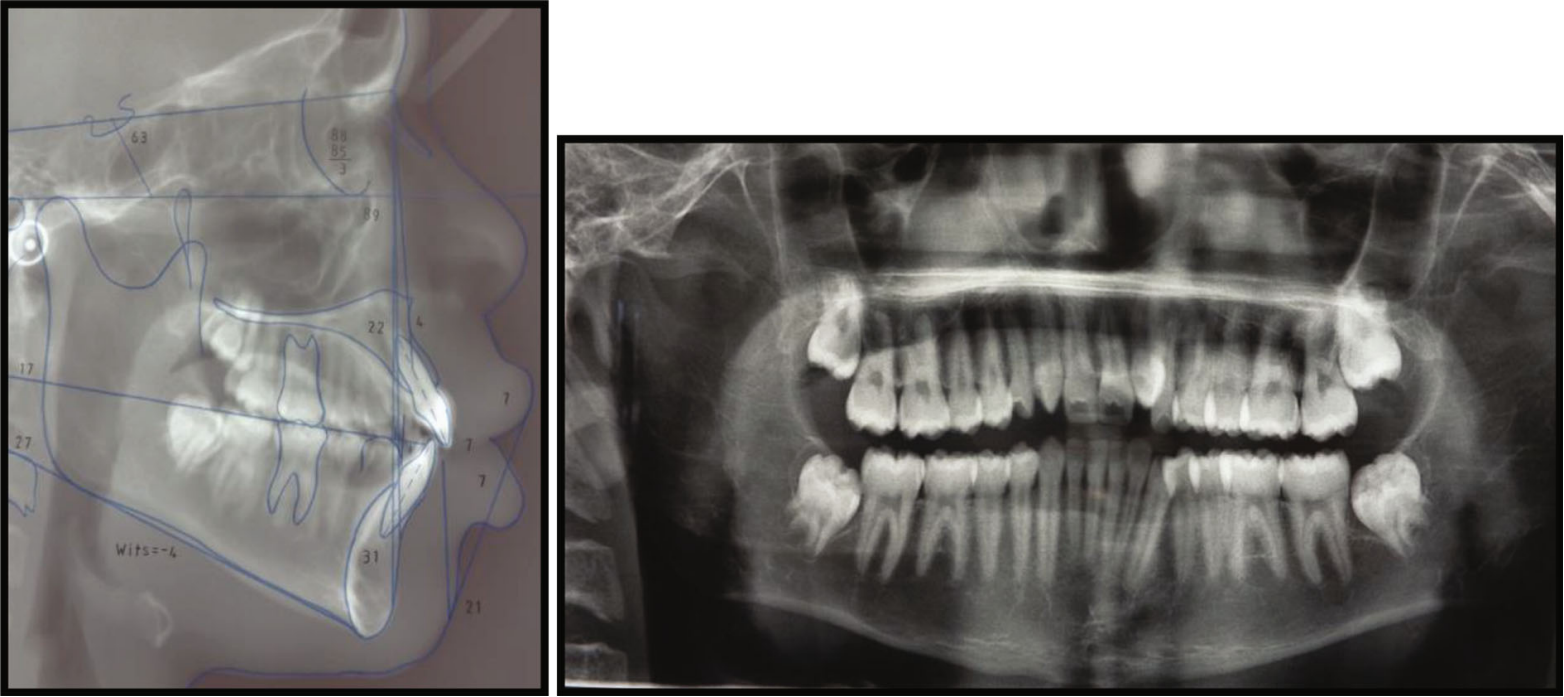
Pretreatment radiographs.
